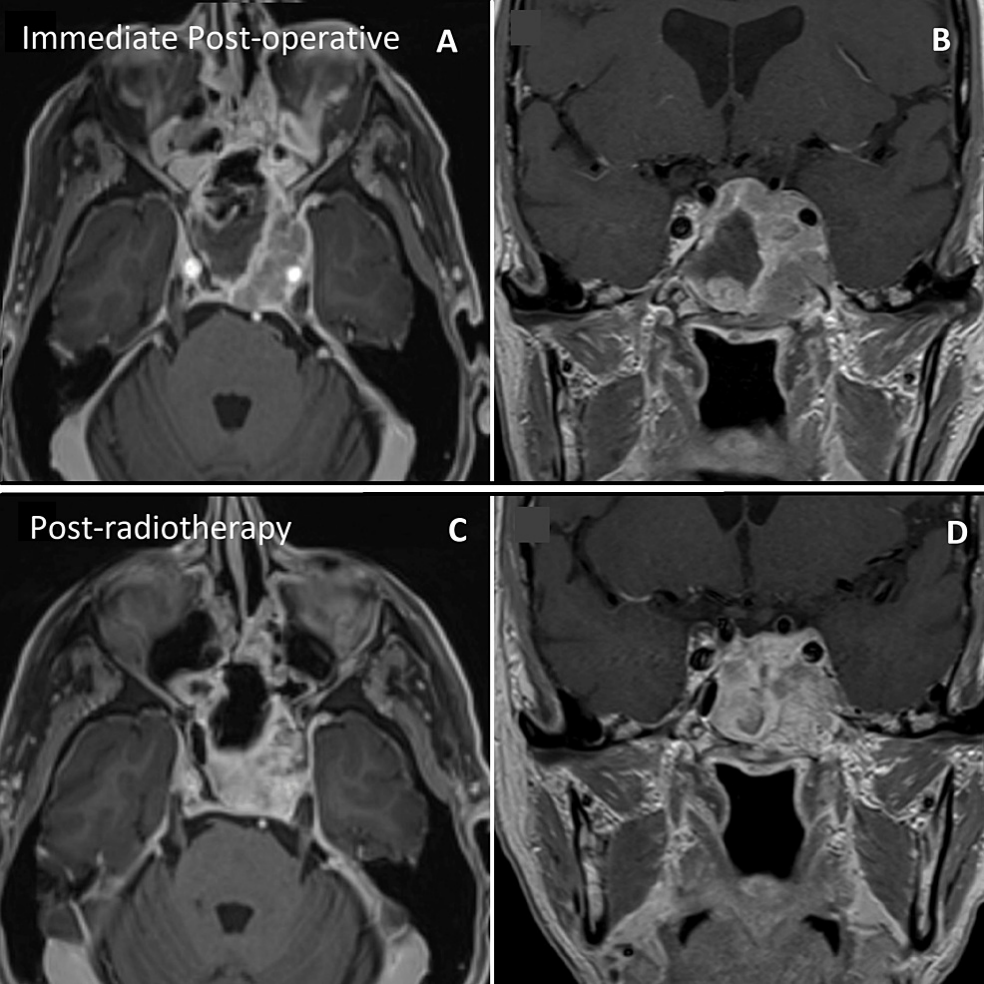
Postoperative and post-radiotherapy imaging A, B: Axial (A) and coronal (B) T1-weighted gadolinium-enhanced MRI showing the postoperative extent of the resection. Approximately 50% of the lesion was resected. C, D: Axial (C) and coronal (D) contrast-enhanced sequences showing the lesion’s partial response to the radiotherapy course MRI: magnetic resonance imaging
